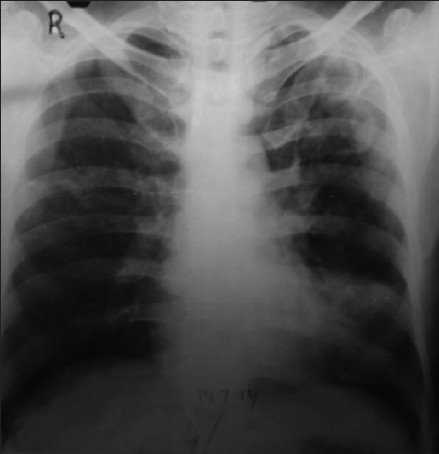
Chest radiograph showing left upper lobe cavitary infiltrate
